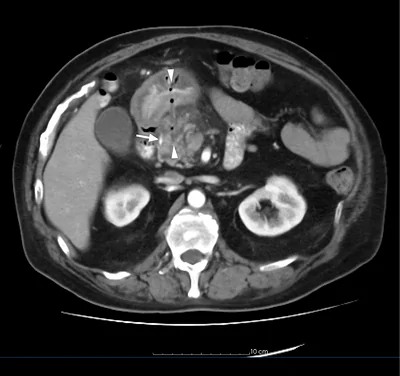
Contrast‐enhanced computed tomography (CT) performed on hospital day 3 for preprocedural vascular assessment, showing that the position of the foreign body was unchanged from the initial study. The two arrowheads indicate the ends of the foreign body, and the arrow indicates the gastroduodenal artery. Although the object lay close to this artery, there was no contact with the celiac artery, superior mesenteric artery, portal vein, or other major vessels, and no active extravasation was seen. Window width, 350 HU; window level, 50 HU. The non‐contrast (Figure 1) and contrast‐enhanced (Figure 2) studies were obtained at different time points and for different purposes—initial diagnosis and preprocedural vascular assessment, respectively—and are therefore presented as separate figures.
